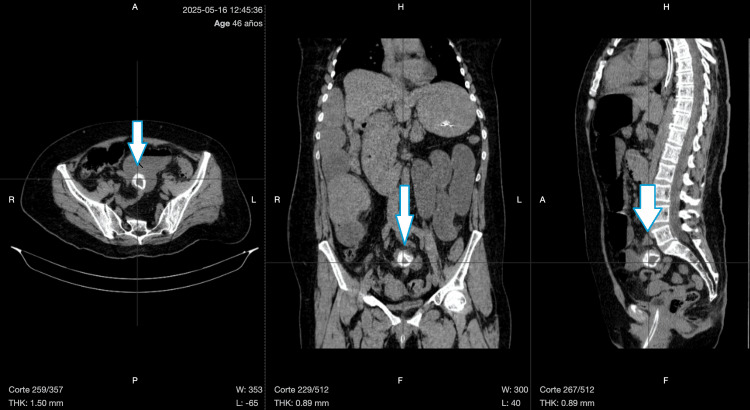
Contrast-enhanced abdominal computed tomography scan in axial (left), coronal (middle), and sagittal (right) planes. The blue arrows indicate a well-defined, hyperdense intraluminal gallstone located in the distal ileum, consistent with mechanical bowel obstruction.
